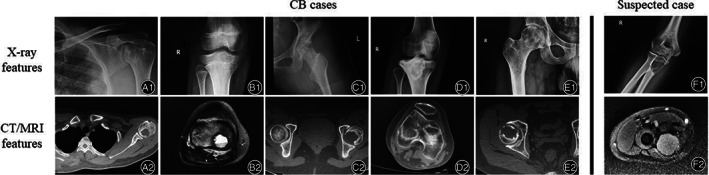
Clinical‐radiological features of CB cases and suspected cases. (A1‐E1) The X‐ray features of CB cases. (F1) The X‐ray feature of suspected case. (A2‐E2) The CT or MRI features of the corresponding CB cases. (F2) The MRI feature of the corresponding suspected case.
